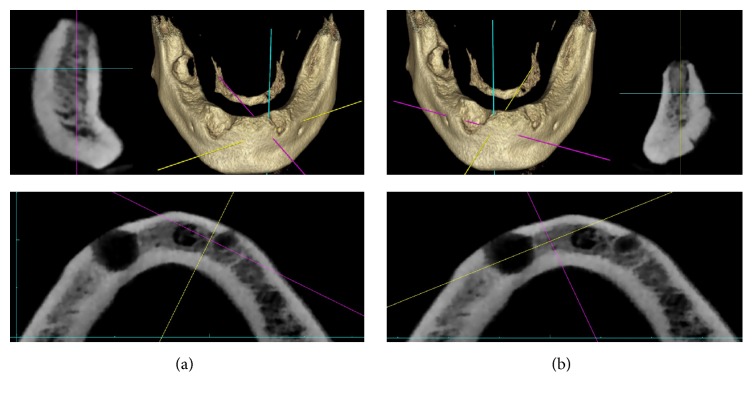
(a) Preoperative CBCT (cross-sectional and axial images) evaluation of the mandibular symphyses after dental extractions. Left mandibular position of the implant. (b) Preoperative CBCT (cross-sectional and axial images) evaluation of the mandibular symphyses after dental extractions. Right mandibular position of the implant.
